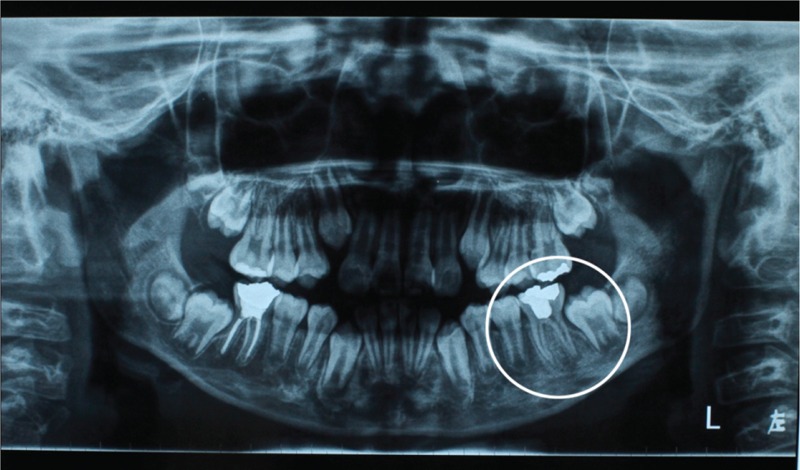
Preoperative radiograph revealed a periapical radiolucent area related to the distal root apex.
